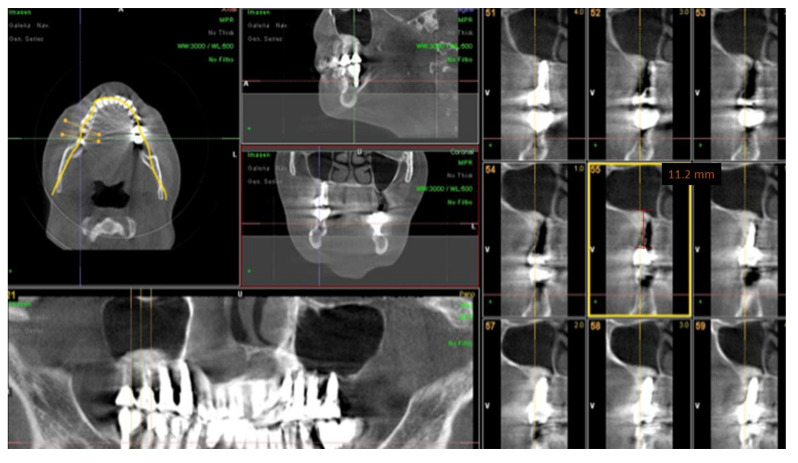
CBCT after maxillary sinus elevation surgery and prosthodontic rehabilitation.
